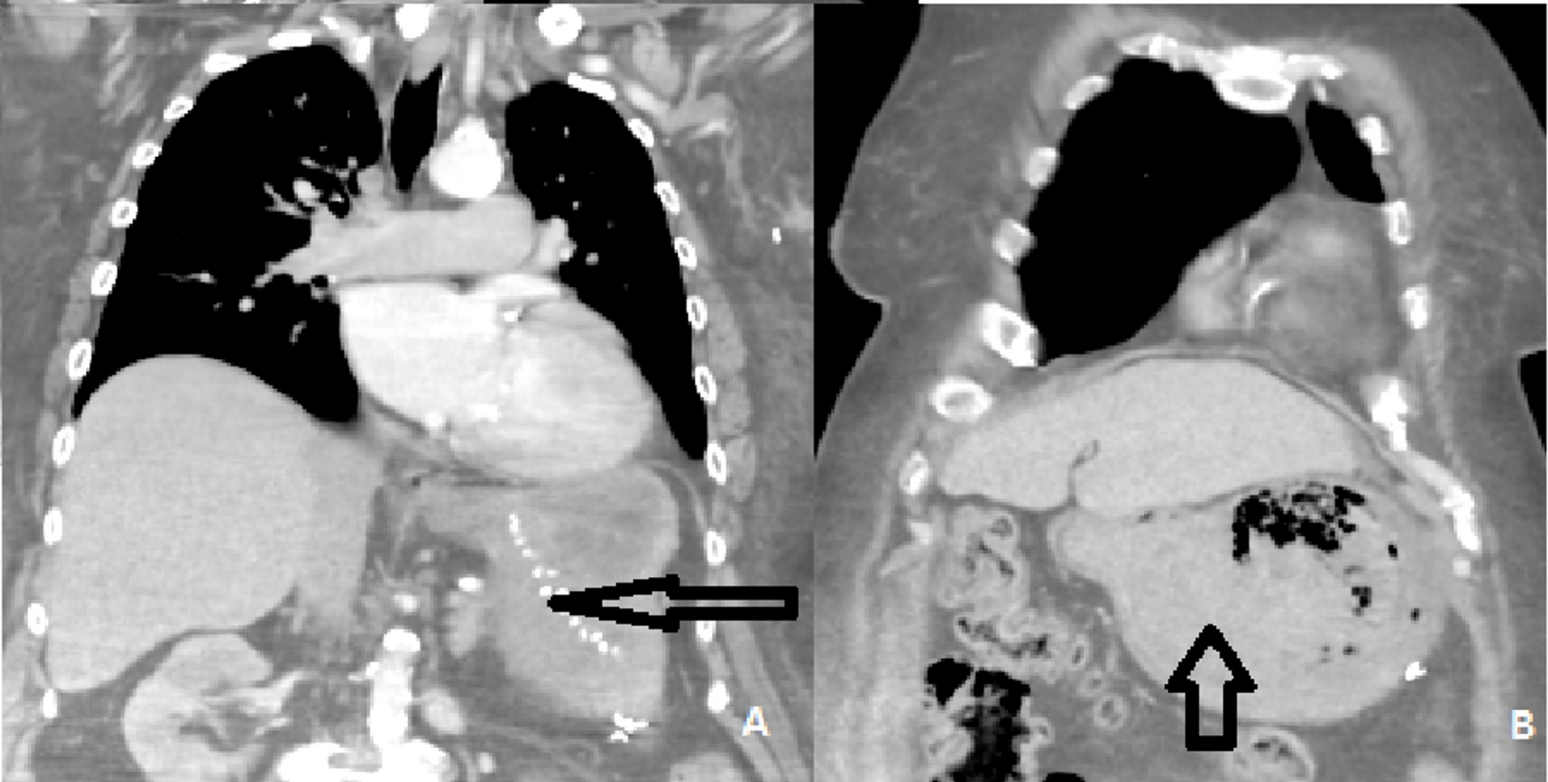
Computed tomography of chest abdomen and pelvis angiography A = arrow showing the evidence of previous gastric bypass B = arrow showing the distended stomach pouch containing blood clot
